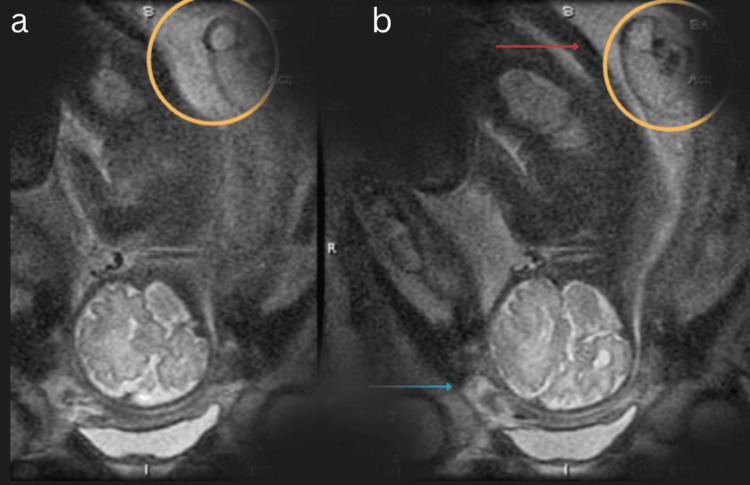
T2-weighted coronal MRI using single-shot fast spin-echo (SSFSE). Views demonstrate a head-forward fetal position, with the blue arrow in panel (b) indicating the face of the pump fetus. The orange circles in panels (a) and (b) highlight an additional inhomogeneous mass adjacent to the fetus’s lower back, indicated by the red arrow in panel (b). Note: This imaging sequence is considered safe in pregnancy, as it is rapid and does not use contrast or ionizing radiation.
